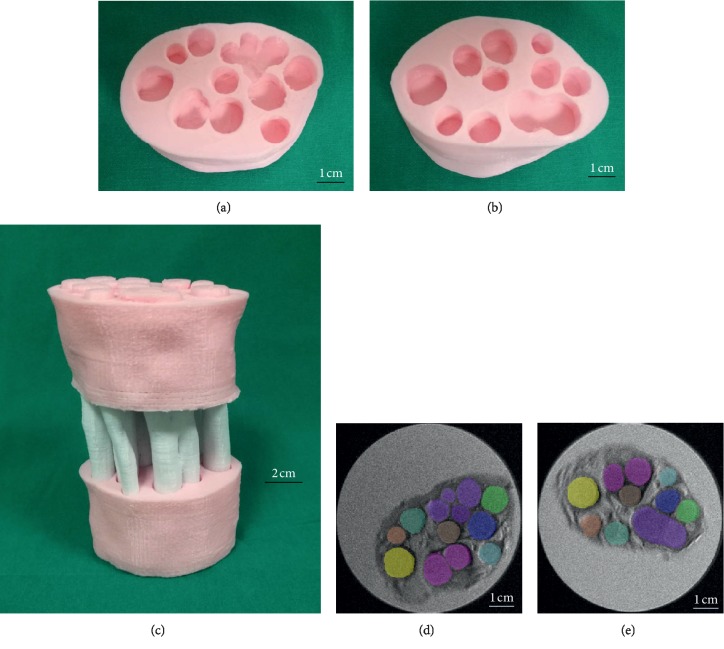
The 3D printing model demonstrates the morphological characteristics of the customized nerve graft that mimics the microstructure of the peripheral nerve fasciculus. (a, b) Significant differences in the number and spatial distribution of the nerve fasciculus in the proximal and distal part of the nerve defect. (c) The 3D printing PLA model demonstrates the morphological features of the customized nerve graft, showing the nerve graft and the proximal and distal parts of the nerve defect are well matched. (d, e) The distribution of the two nerve tracts corresponding to the original Micro-MRI scan image. (a, b) Scale bar 1 cm. (c) Scale bar 2 cm. (d, f) Scale bar 1 mm.
